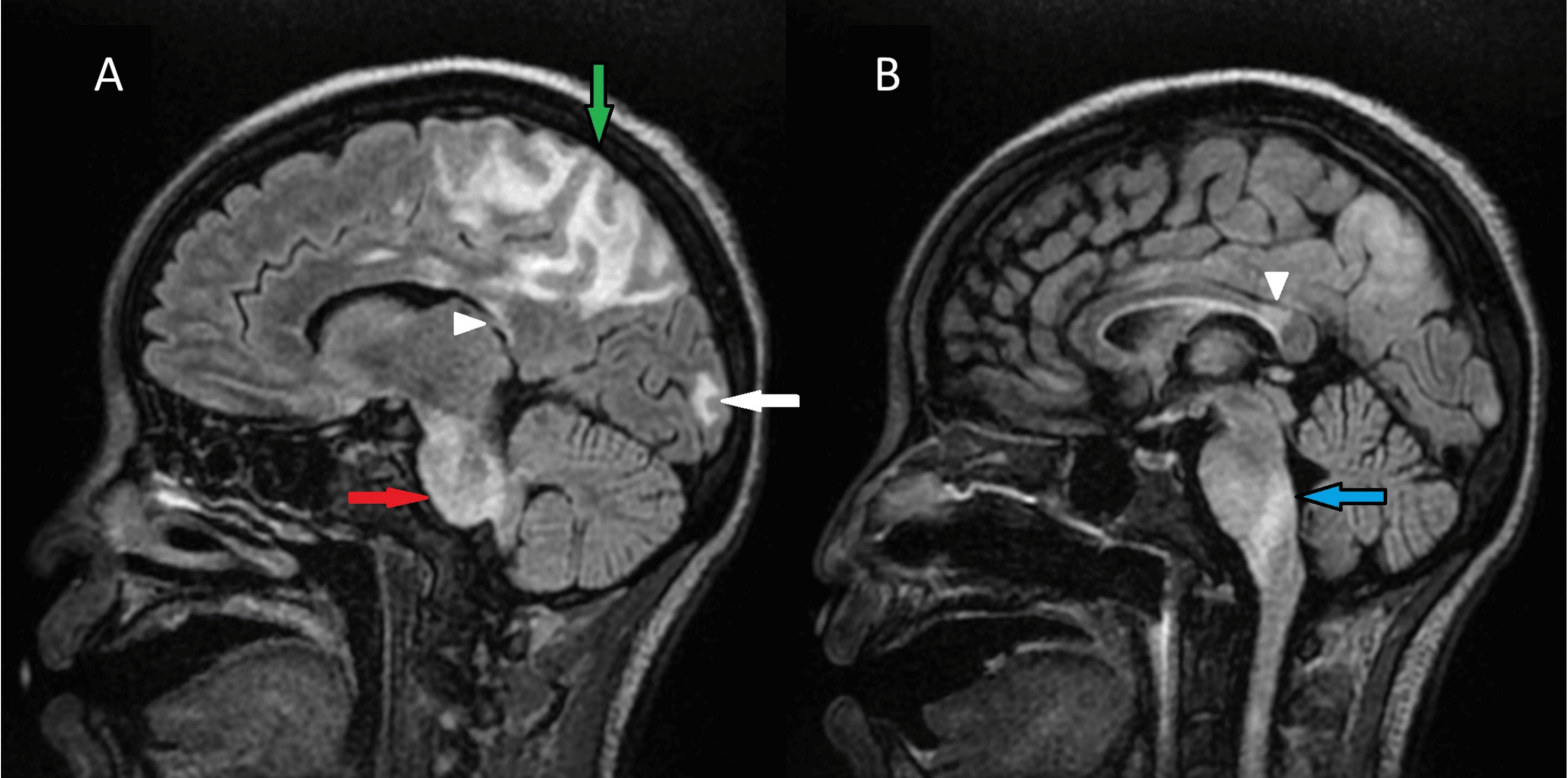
Left parasagittal FLAIR image demonstrates white matter hypointensities involving the parietal lobe (green arrow), occipital lobe (white arrow), corpus callosum (arrowhead), medulla (blue arrow), and pons (red arrow) (A). Sagittal FLAIR image better demonstrates involvement of the callosal isthmus (arrowhead) and medulla (blue arrow) (B).
